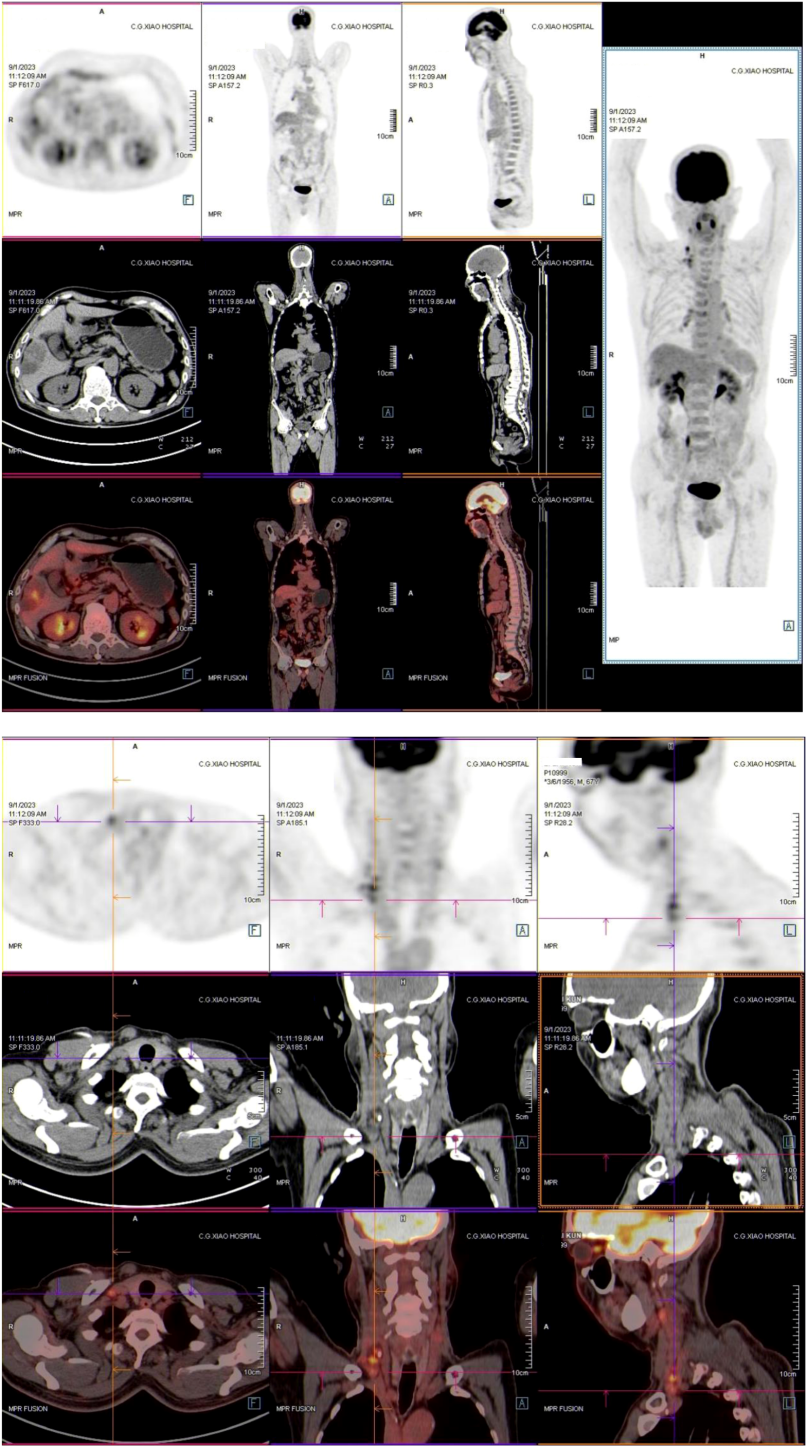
Full body PET-CT images of the patients in September 2023.
